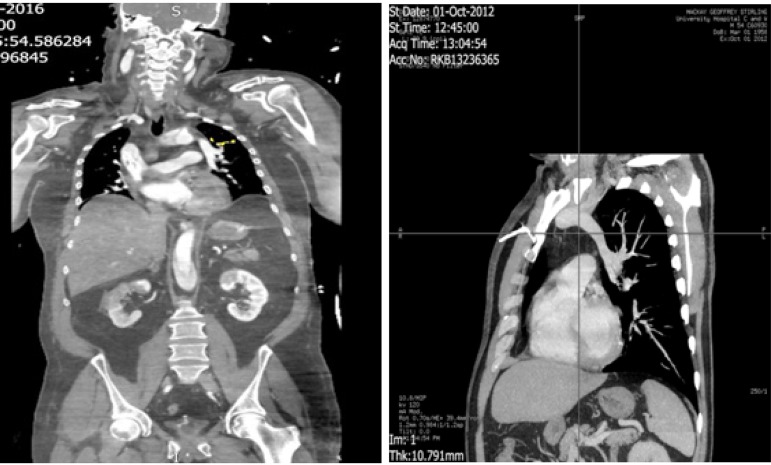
The contrast CT scan showing anomalous left superior pulmonary vein.
